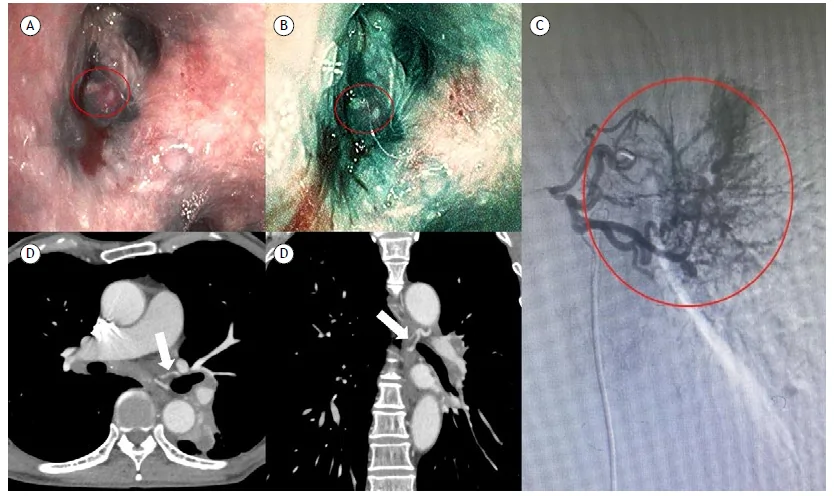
In A, white-light bronchoscopy revealing a congested, tumor-like protrusion (red circle) in the proper segment of the upper lobe. In B, narrow-band imaging revealing dilated submucosal vessels appearing in dark bluish green (red circle) and corresponding to the smooth protrusion seen under white light; by enhancing mucosal-vascular contrast, narrow-band imaging supports the diagnosis of submucosal arterial malformation. In C and D, axial and coronal contrast-enhanced CT scans showing the dilated bronchial artery (arrows) protruding into the bronchial lumen. In E, digital subtraction angiography showing the catheter tip just entering the main trunk of the bronchial artery, with the filled vascular malformation cluster (red circle) visible at the instant before embolization.
